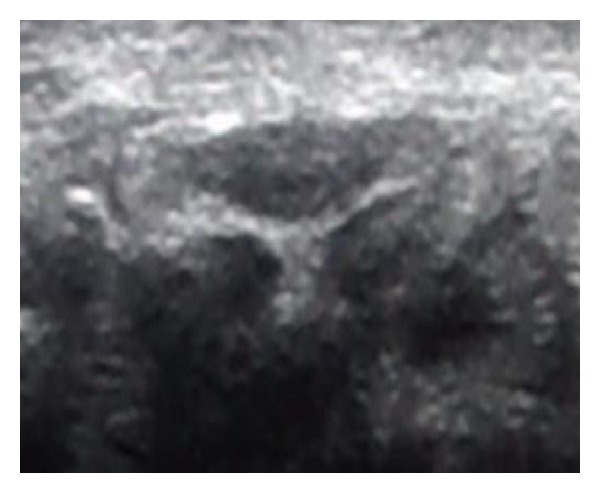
Wrist ultrasound at the carpal tunnel inlet for patient with moderate CTS, the cross sectional area of the median nerve was 14 mm^2^.
